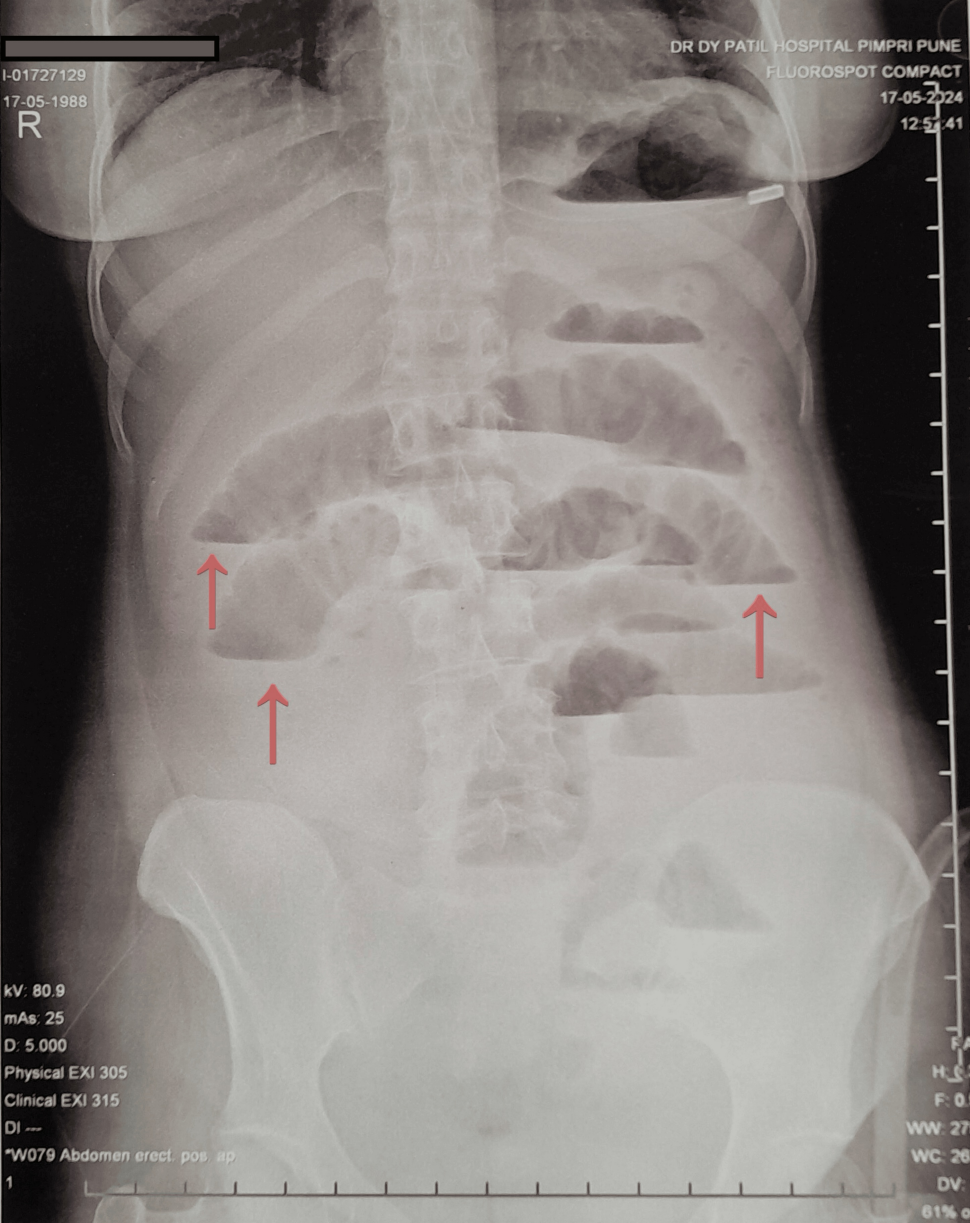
Erect abdominal X-ray showing multiple air-fluid levels (red arrows), suggestive of small bowel obstruction.
